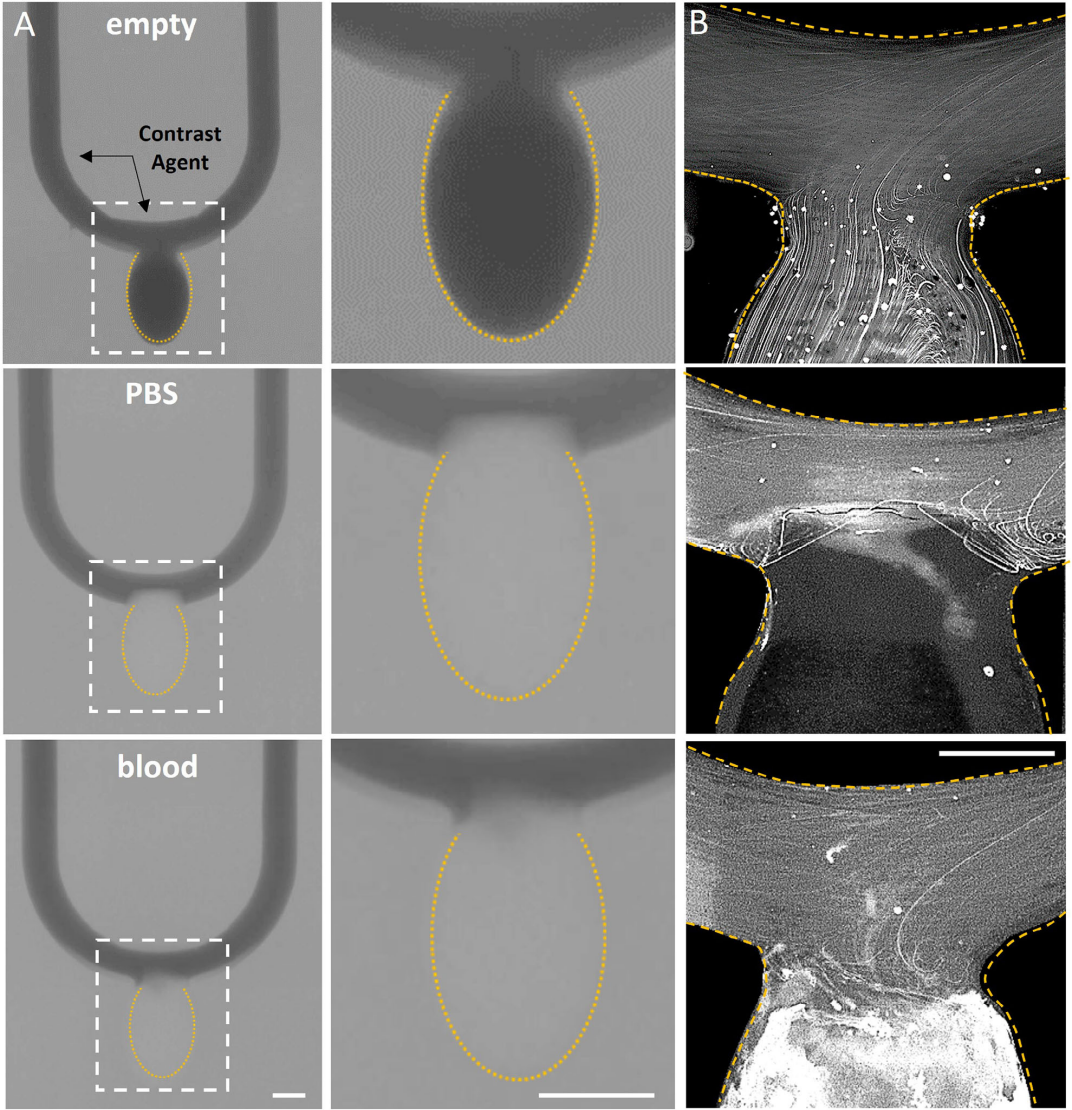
Unidirectional swelling of GAG analog hydrogels in a 3D printed aneurysm model under flow. A) CT images of 3D aneurysm model, either empty (no hydrogel, top) or embolized with 4% hydrogels in the presence of PBS (middle) or the presence of human blood (bottom), all under physiological flow. Scale bar (right and middle columns): 3 mm; B) Respective pathlines of 10 µm fluorescently labeled polystyrene particles under physiological flow. Scale bar: 1.5 mm.
